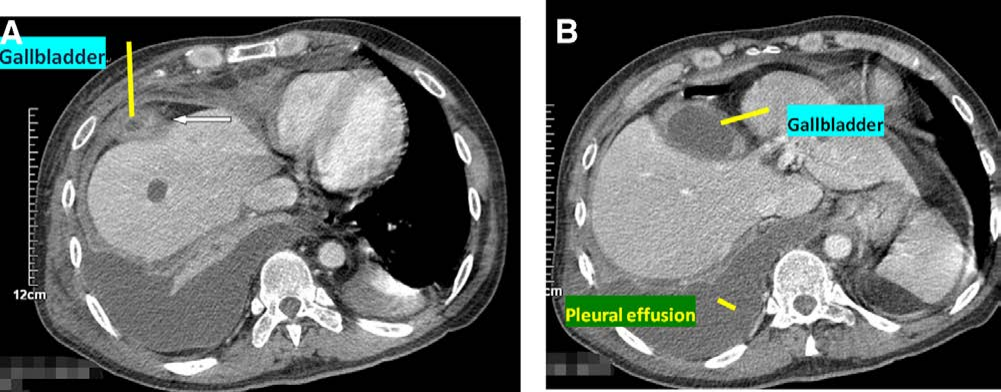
(A and B) Computed tomography showed cholecystitis and peri-inflammation with a small abscess around the base of the gallbladder, local peritonitis, a small amount of fluid in the abdominal cavity, and bilateral pleural effusion. The white arrow indicates a small abscess around the gallbladder. The yellow oblique line indicates cholecystitis with pericholecystitis.
